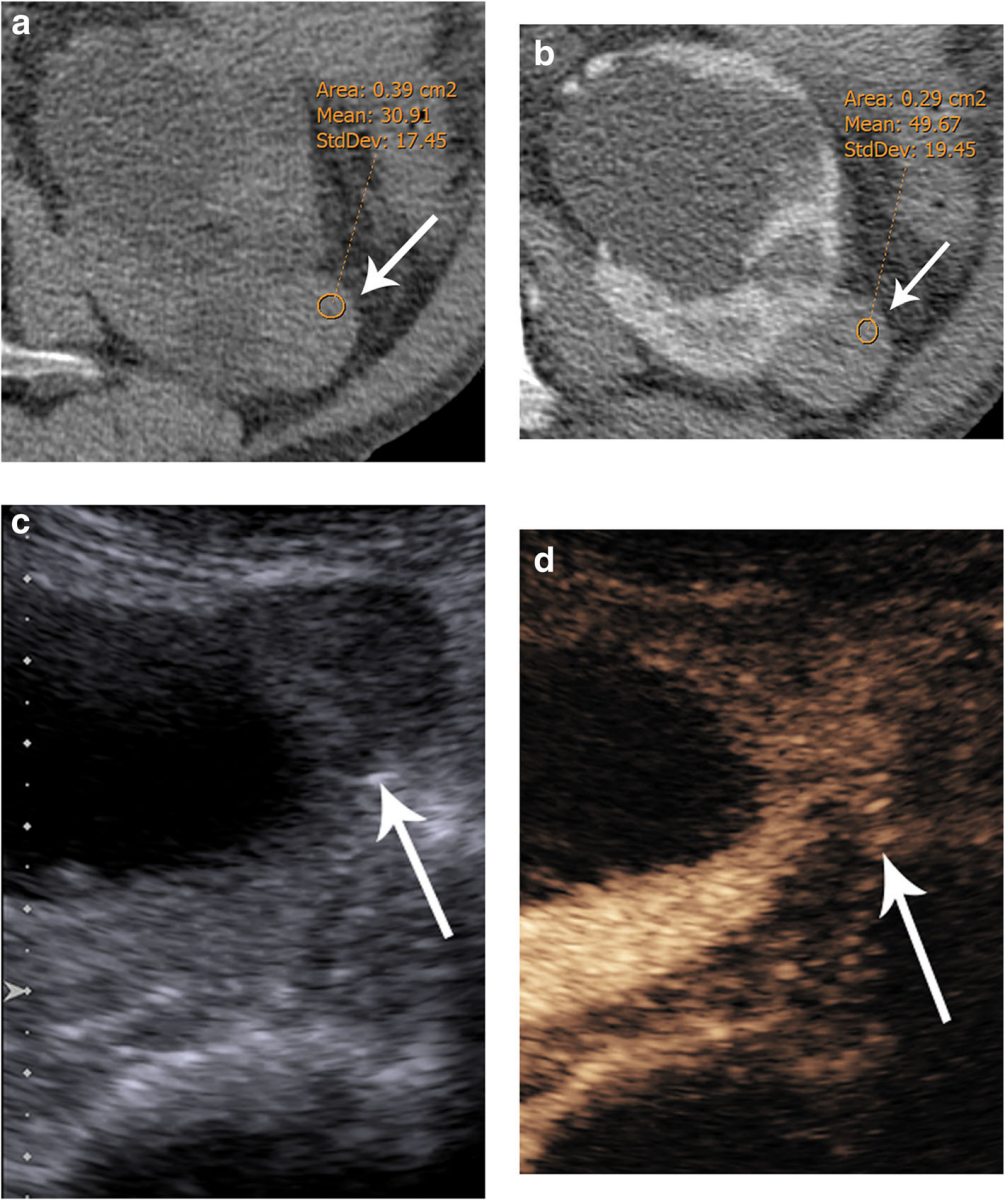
Indeterminate renal mass by CT in an 82-year-old patient. a Axial unenhanced and (b) enhanced CT showing a renal mass with attenuation greater than 20 HU in unenhanced CT that enhances less than 20 HU after contrast administration, considered indeterminate by CT (arrows). c Unenhanced and (d) contrast-enhanced ultrasound (US) of the same lesion clearly depicting enhancement of this lesion consistent with a solid renal tumor (arrows), thereby allowing an indeterminate CT renal mass to be reliably classified as a solid mass
